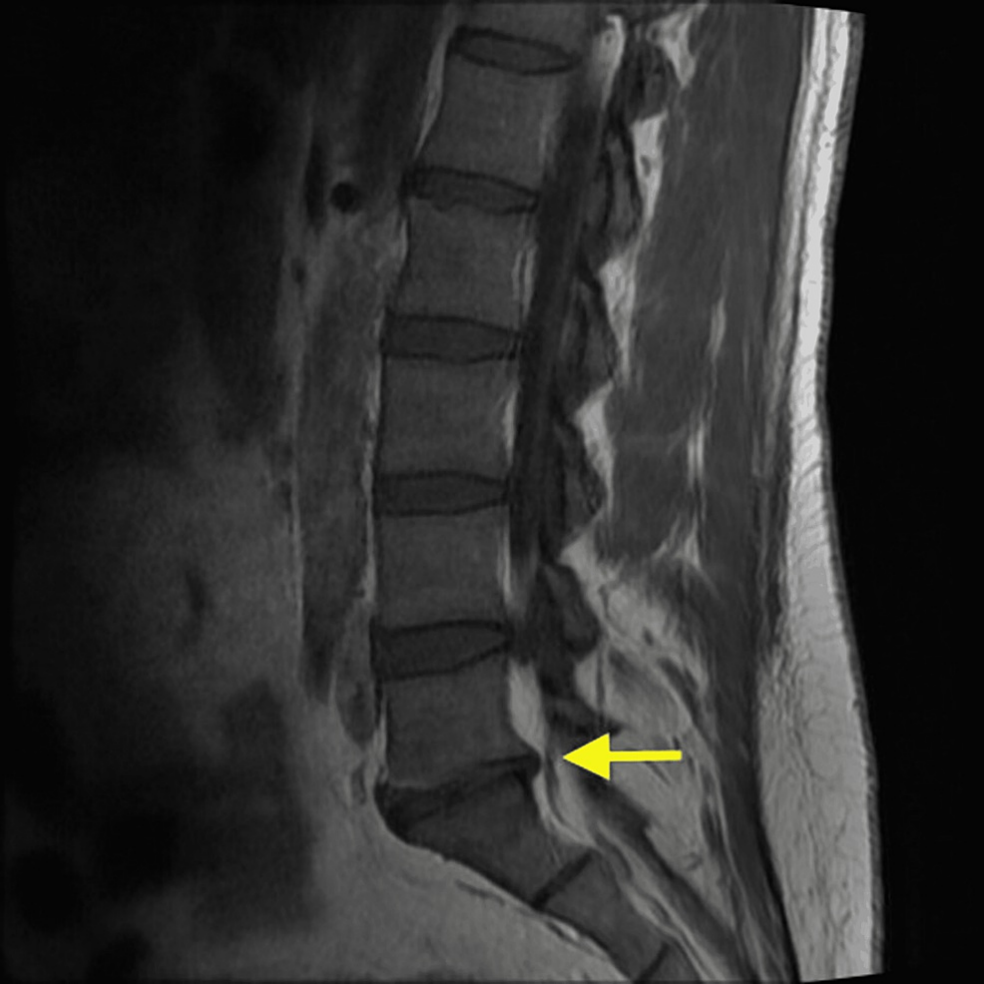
T1-weighted MRI lumbar spine without contrast demonstrated L5-S1 disc space narrowing with posterior disc bulge.
